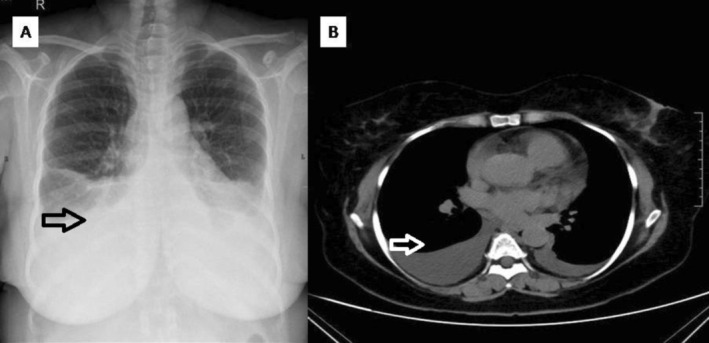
Case 4 (A) Chest radiograph showing bilateral costophrenic angle blunting. (B) Contrast‐enhanced CT showing right moderate and left pleural effusion.
